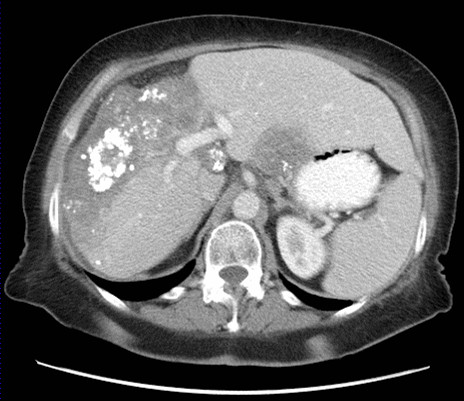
Computed tomography scan of the abdomen performed in 2009 demonstrating an unchanged size of the liver lesion over the seven-year period but with the development of calcification.
